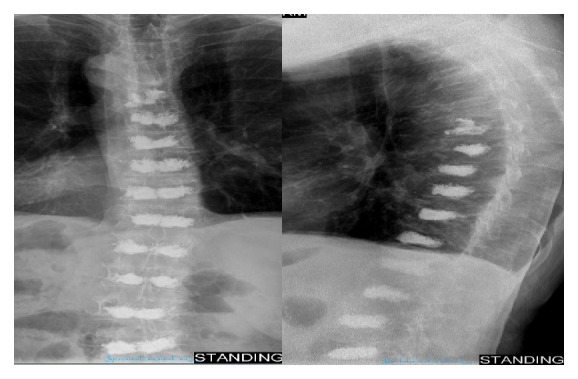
AP and lateral X-rays taken of the thoracic spine following the last of the eleven kyphoplasties. The patient underwent two kyphoplasties of T8 and one each of T9–T12.
